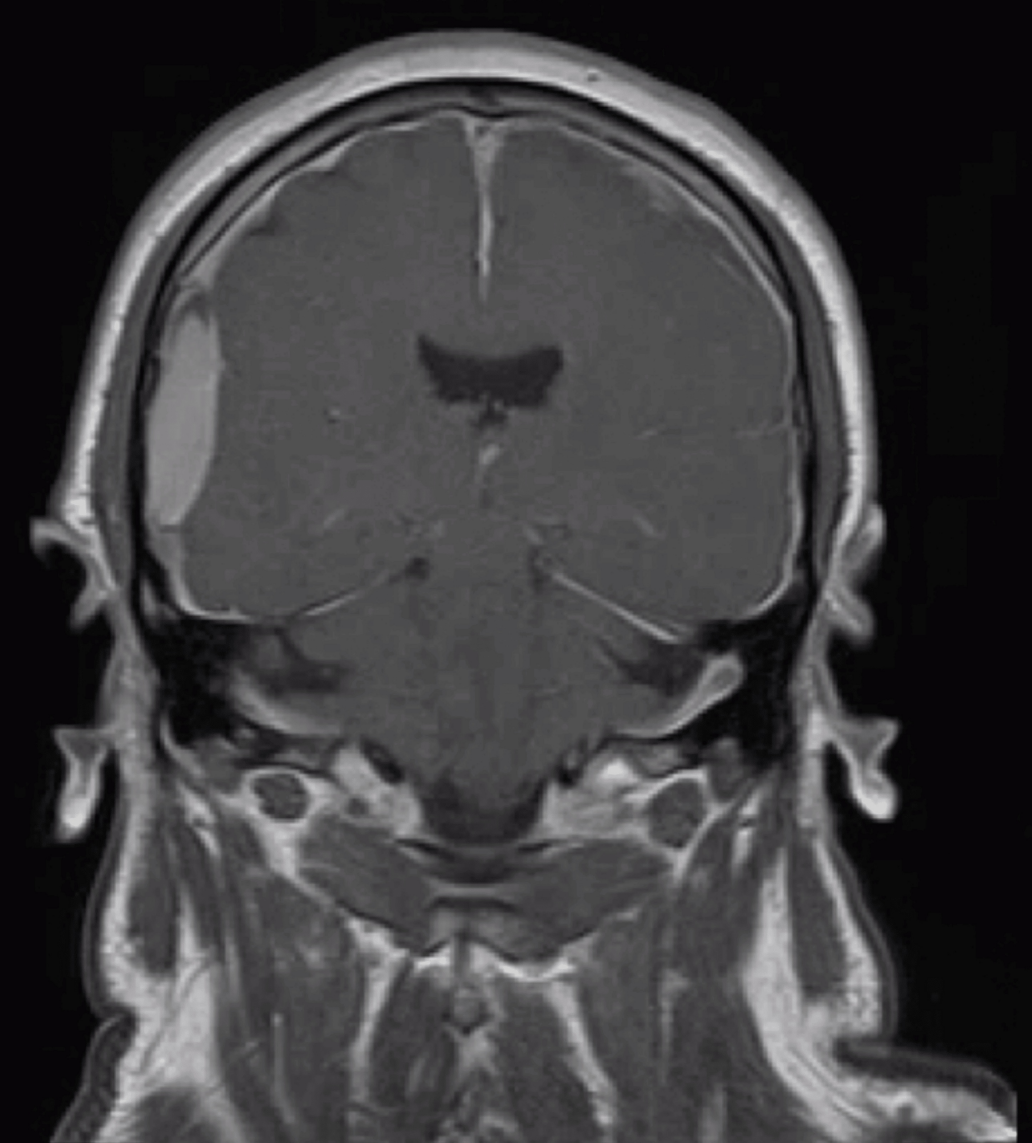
MRI Brain Following Hemicraniectomy MRI brain T1 post gadolinium contrast reveals right SDH with appearance of diffuse leptomeningeal enhancement indicative of spontaneous intracranial hypotension MRI - Magnetic Resonance Imaging SDH - Subdural Hemorrhage
